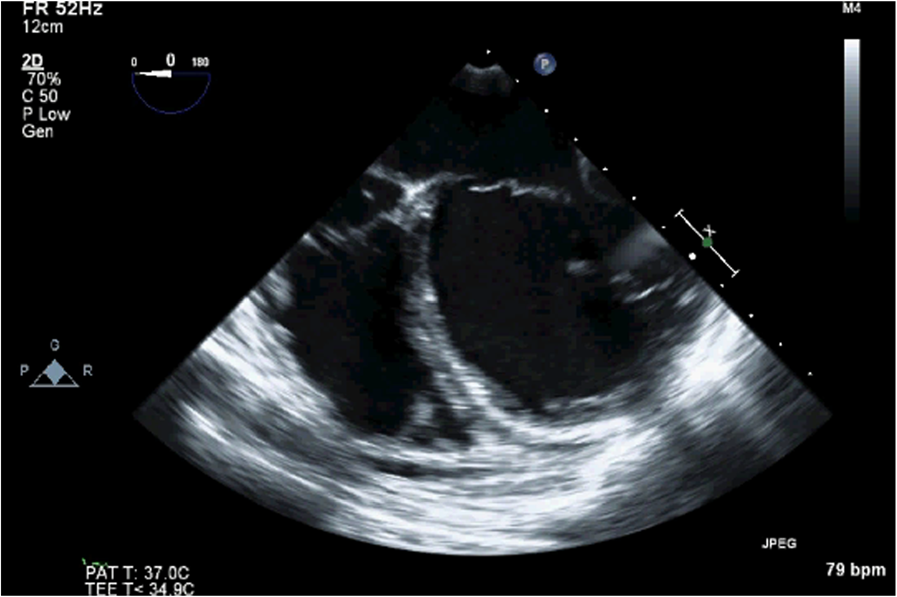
A transesophageal echocardiographic image. Mid-esophageal 4-chamber view showing the severely dilated right and left ventricles. The intraventricular septum was bowing into the right ventricle
